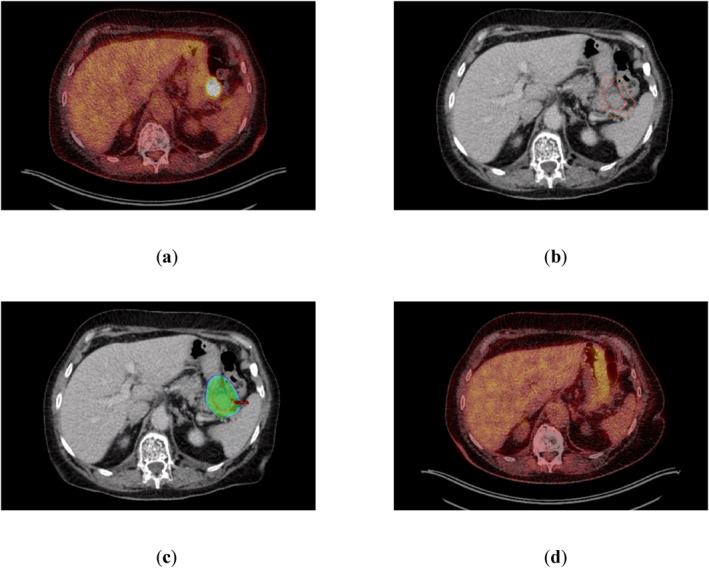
Salvage radiotherapy (RT) for a 63‐year‐old and heavily pretreated woman under ST with teclistamab presenting a paragastric lesion on a Positron emission tomography‐computer tomography (PET‐CT) scan. No additional lesions were detected. The interdisciplinary tumor board recommended local RT with continuation of teclistamab. (a) PET‐CT scan demonstrating a hypermetabolic paragastric lesion (standard uptake value 9.7; Deauville‐Score 5). (b) Planning‐CT indicating the PET‐CT‐based Gross Tumor Volume (red) and Planning Target Volume (orange). (c) 95%‐isodose as color‐wash dose distribution based on a 6 MV intensity‐modulated‐radiotherapy‐plan using 5 fields (prescribed dose 30.6 Gy in fractions of 1.8 Gy) (d) PET‐CT scan 90 days after completion of RT demonstrating a complete metabolic remission. Sustained serological complete remission was documented 6 months after completion of RT.
